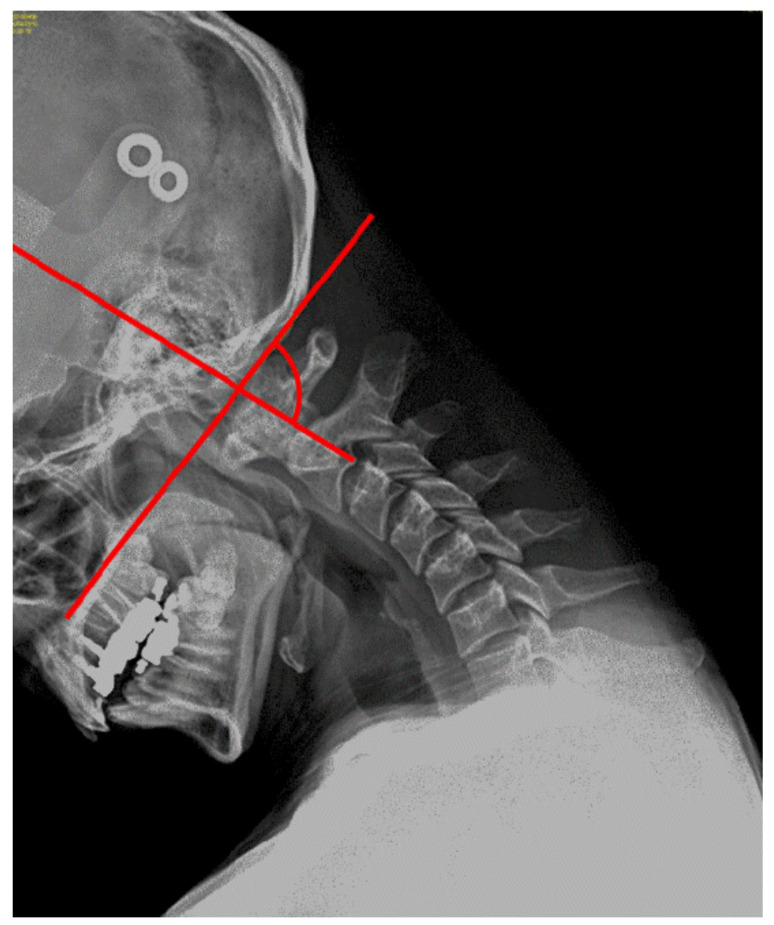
Measurement of upper cervical flexion angle using X-ray.
